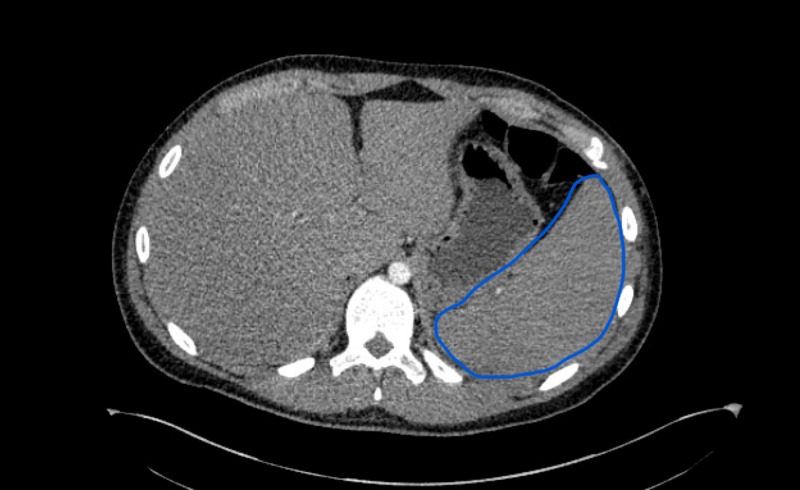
CT Chest Blue outline indicates splenomegaly
